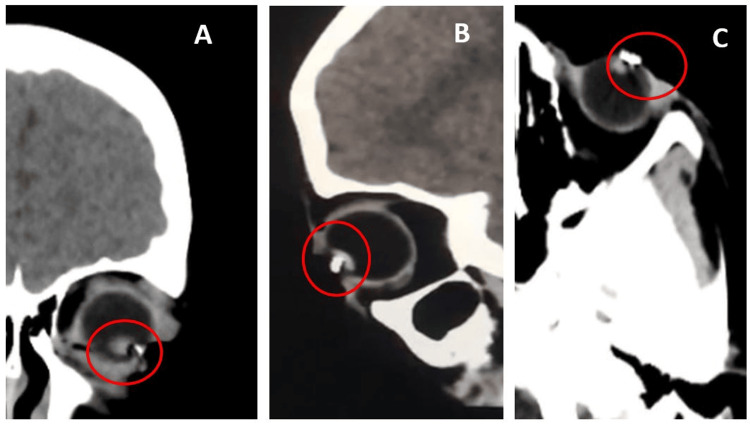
Head CT Scan Series A. Coronal view. B. Sagittal view. C. Axial view showing a metallic foreign matter within the left globe extending to the anterior chamber (red circle).
